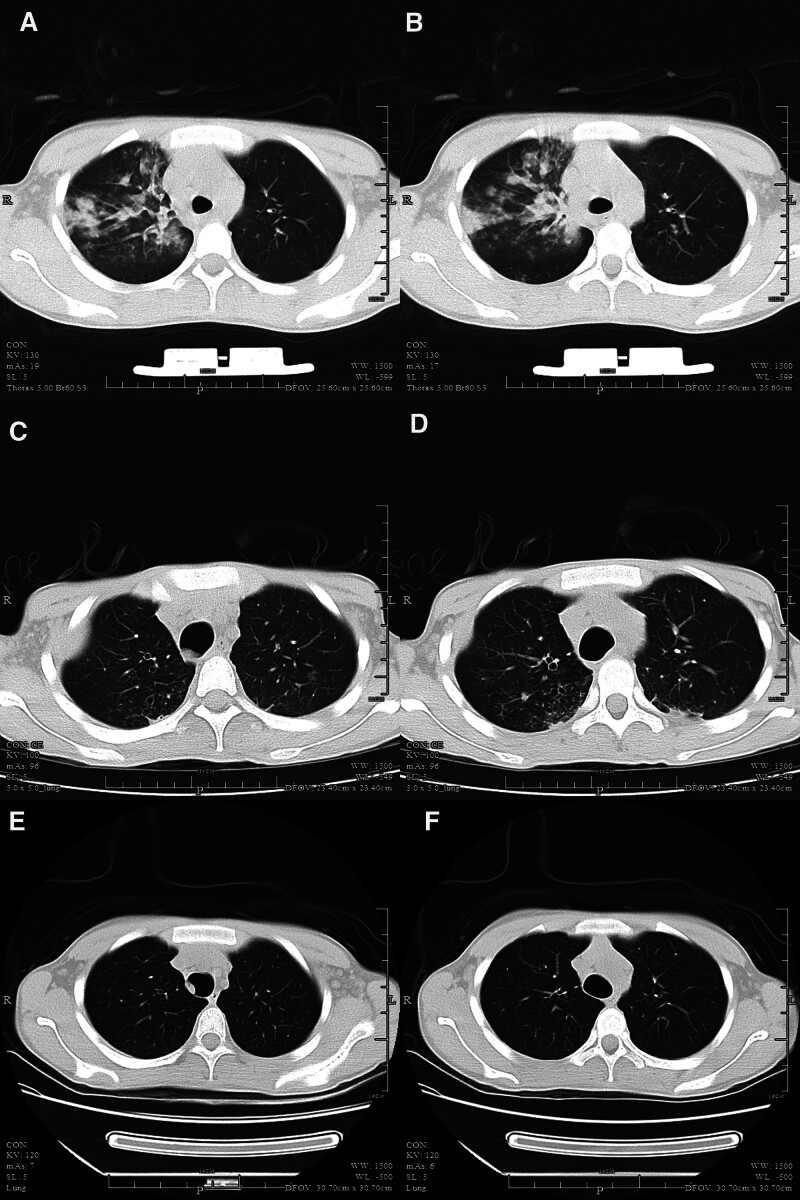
Imaging (A, B) February 24, 2022 chest CT revealed multiple infiltrations in both lungs. (C, D) April 25, 2022, chest CT showed less multiple infiltration in both lungs. (E, F) February 12, 2023 chest CT showed exudation lesions in both lungs accompanied by a few cystic shadows, which were smaller than before. CT = computed tomography.
